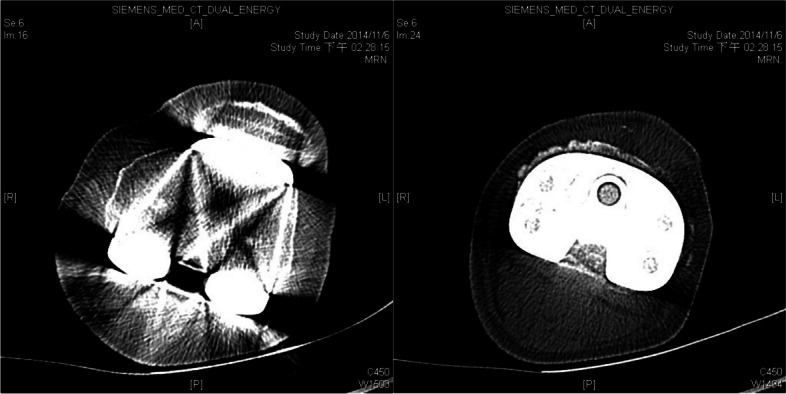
Absence of malrotation of femoral and tibial components through preoperative computed tomography
